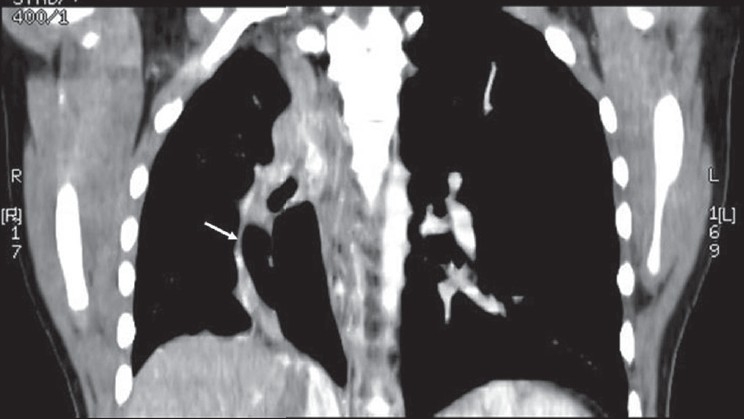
CT thorax showing a curved linear (scimitar shape) opacity running from the middle of the lung toward diaphragm (shown as arrow)
